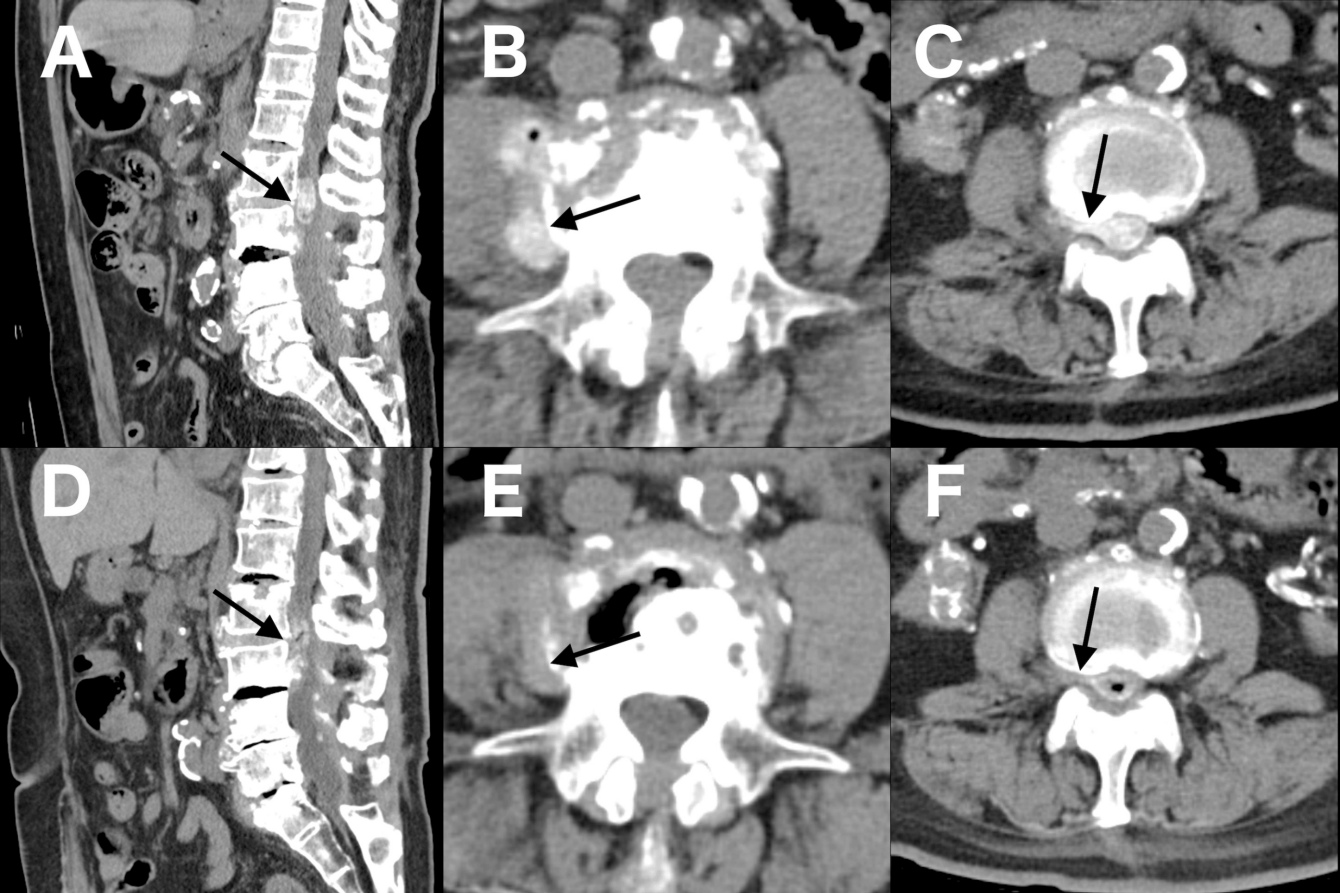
CT Images on day 28 and follow-up CT findings two months after discharge A-C: CT images on day 28 of admission, after removal of drainage tube, showing residual epidural and perivertebral calcifications (black arrows). The perivertebral calcification in panel B was the site of the biopsy performed on day 15. D-F: CT images at the two-month follow-up obtained at the same levels as panels A, B, and C (taken on day 28), respectively, demonstrating the regression of epidural calcification. Notably, the perivertebral calcification visible in panel B disappeared in panel E (black arrows).
